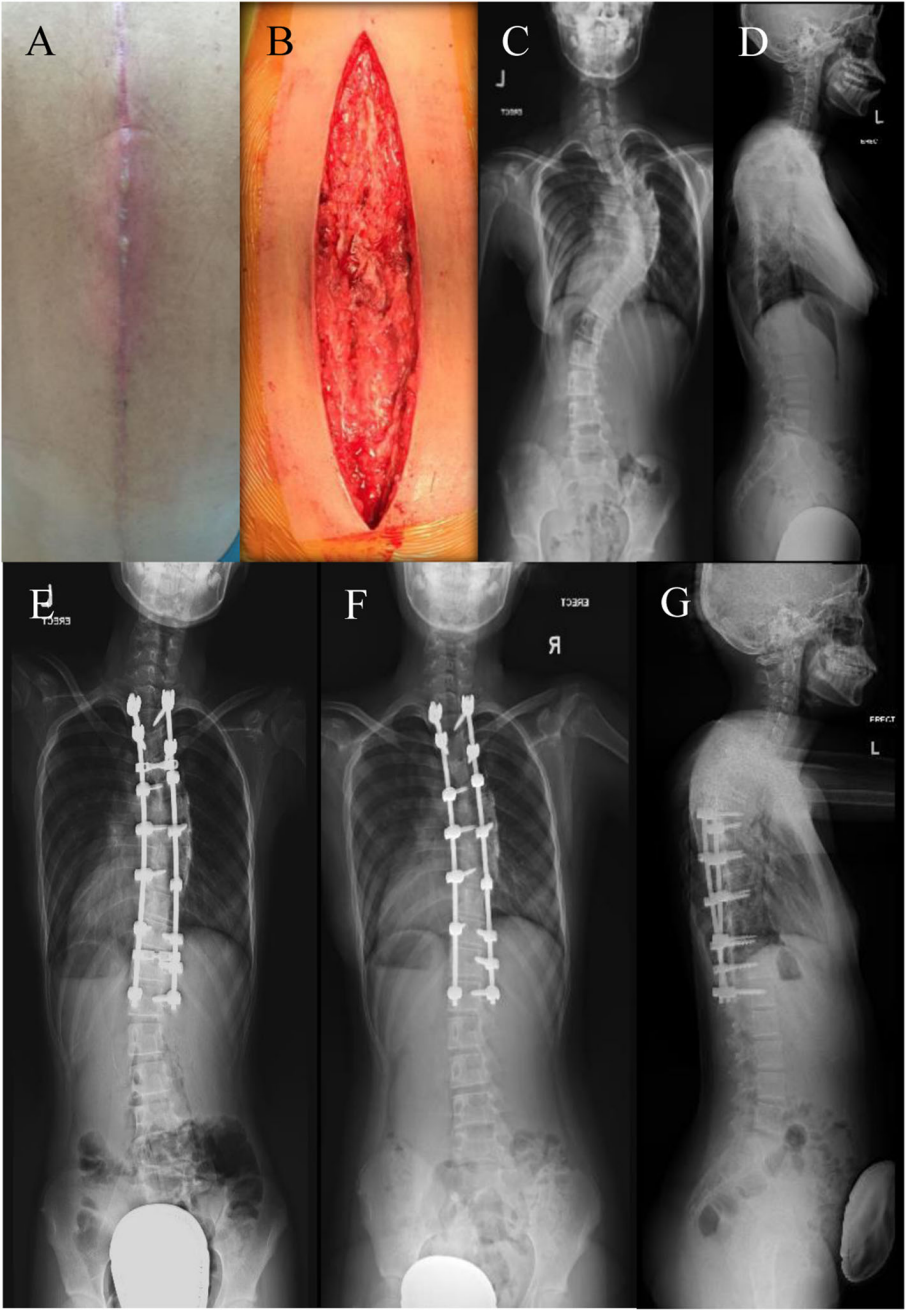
A patient developed deep surgical site infection 3 weeks postoperatively; a showed preoperative swelling and redness over surgical site; b was post-debridement intraoperatively). c & d were preoperative and e was postoperative AP radiograph. She was treated successfully with early debridement and removal of crosslinks within 24 h of presentation (f & g)
